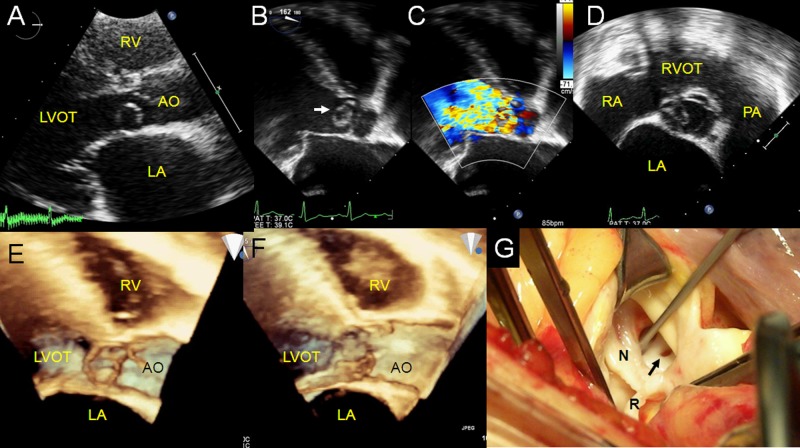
An aneurysm of the non-coronary sinus of Valsalva that has ruptured into the left ventricle. A saccular lesion extends into the left ventricular outflow tract, but we could not determine from transthoracic echocardiographic (TTE) scans whether it had ruptured or not (A). Transesophageal echocardiography (TEE) reveals a small aneurysmal defect (arrow) (B), the flows from the defect, the aortic valve (C), and the aneurysmal origin (D). We used real-time 3D TEE to further characterize the steric morphological changes during the cardiac cycles (E, F). An intraoperative photograph shows that the aneurysm (indicated by the probe in the middle of the picture) communicated with the left ventricle through the defect at its tip (arrow), viewed from the aortic root (G). AO: aorta; LA: left atrium; LVOT: left ventricular outflow tract; N: non-coronary cusp; PA: pulmonary artery; R: right coronary cusp; RA: right atrium; RV: right ventricle; RVOT: right ventricular outflow tract.
